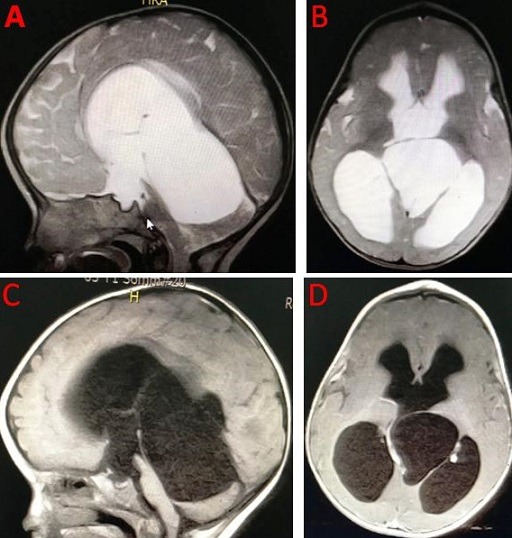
Magnetic resonance image (MRI) demonstrated enlargement of the supratentorial ventricular system secondary to a large quadrigeminal cistern arachnoid cyst compressing the brainstem, cerebellum, aqueduct of sylvius and fourth ventricle. The cystic mass appeared hyperintense on T2 weighted-images (A, B), hypointense on T1-WI (C, D), similar to cerebrospinal fluid (CSF) signal, without enhancement after Gadolinium administration
